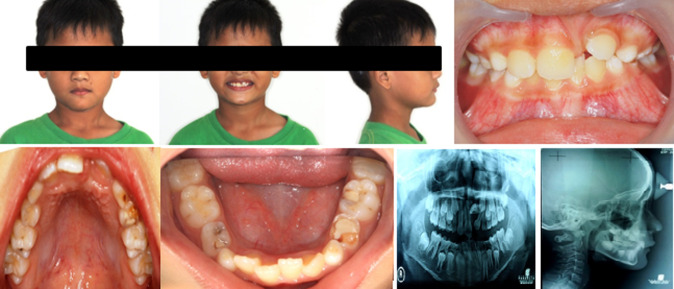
pre-treatment profile, intra-oral, and radiograph view
